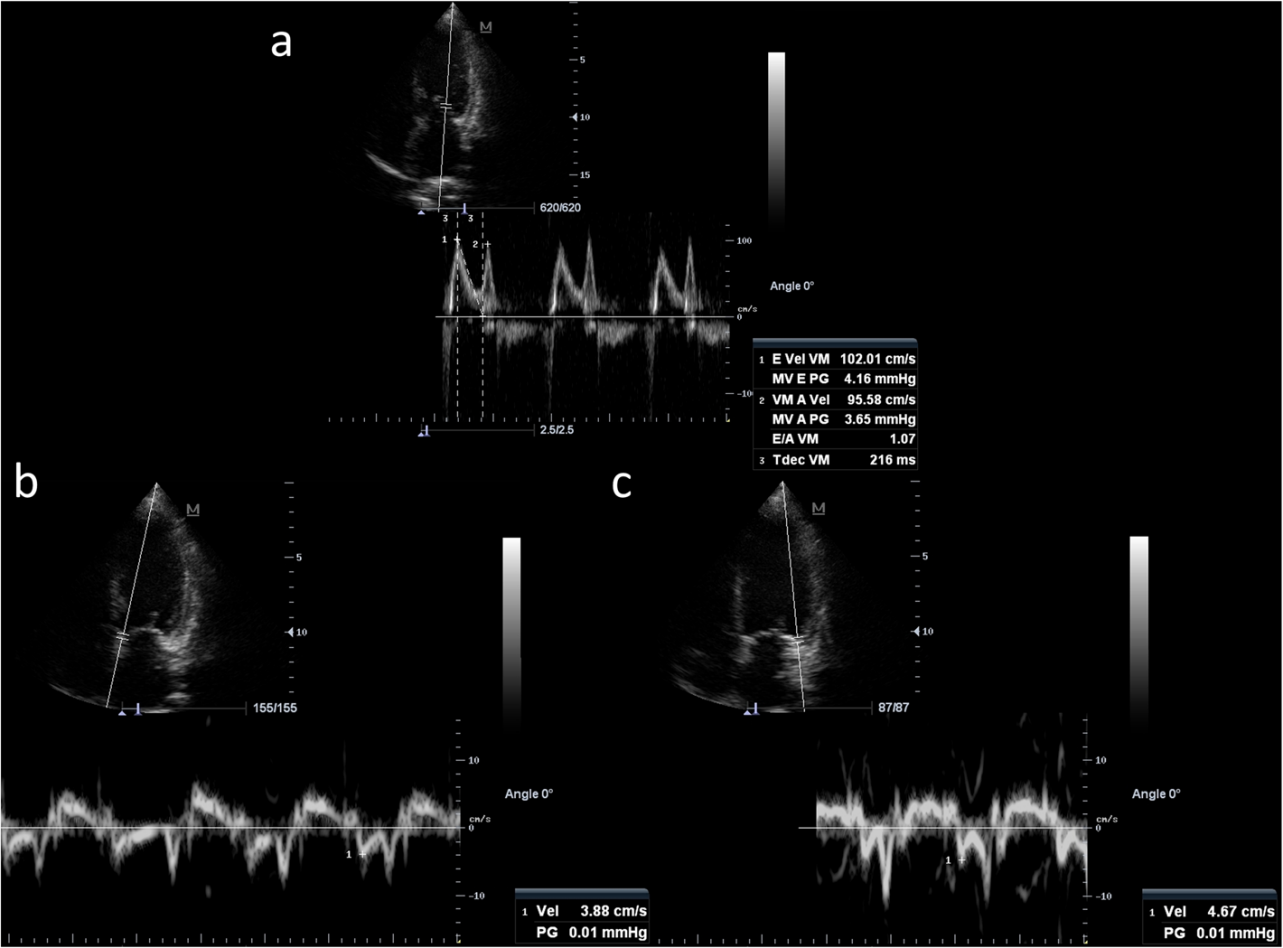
Example for estimating the left ventricle filling pressures from transmitral flow (a) and mitral annular velocities (septal in b and lateral in c), obtained in apical four-chamber view. The E velocity is 102 cm/s and the averaged annular velocity (septal and lateral/2) is 4.27 cm/s, with E/Ea ratio of 23.8, indicating a severe elevation of filling pressures
